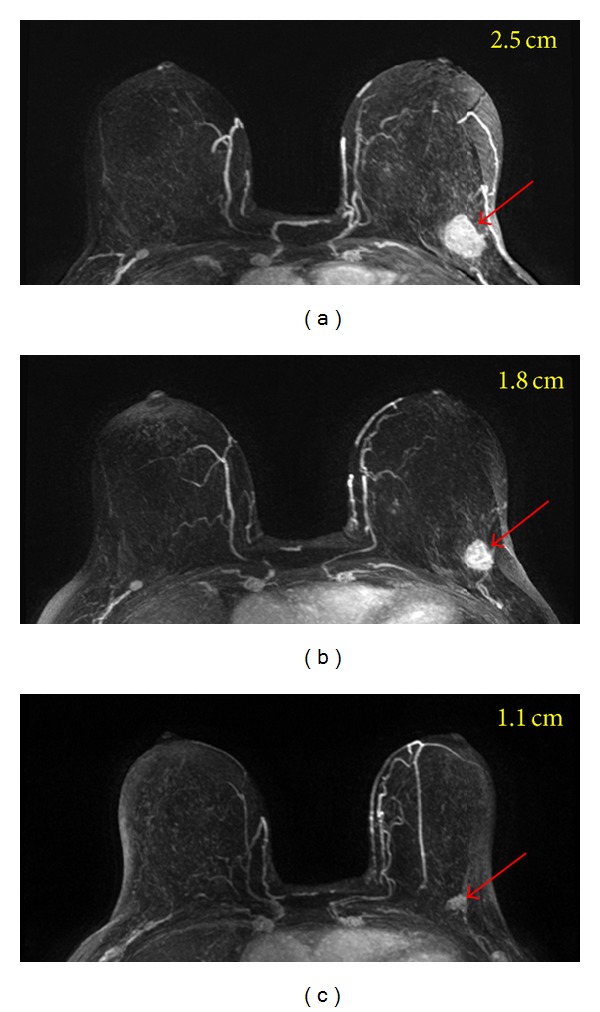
A 64-year-old patient with a well-circumscribed mass lesion (invasive ductal cancer) in the left breast. From (a) to (c), the maximum intensity projection (MIP) images of pretreatment, F/U-1, and F/U-2 MRI are shown. The tumor size is 2.5 cm before treatment and shows concentric shrinkage to 1.8 cm in F/U-1 and further down to 1.1 cm in F/U-2 after completing treatment. The residual tumor size determined in post-NAC pathological examination is 1.4 cm. For mass lesion that shows concentric shrinkage, MRI is accurate in diagnosing residual disease.
